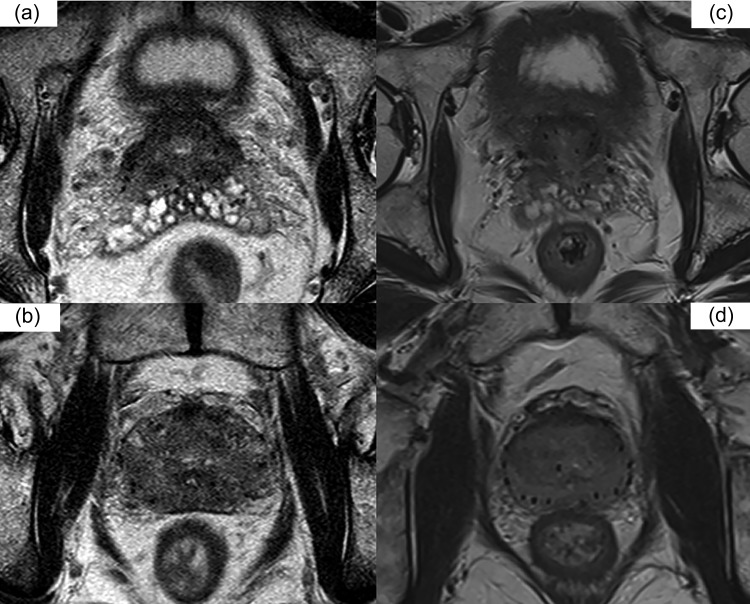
Comparison of the images obtained with 1.5-T magnetic resonance imaging (MRI) and 3.0-T MRI systems. (a, b) 1.5 tesla MRI, (c, d) 3.0 tesla MRI.
